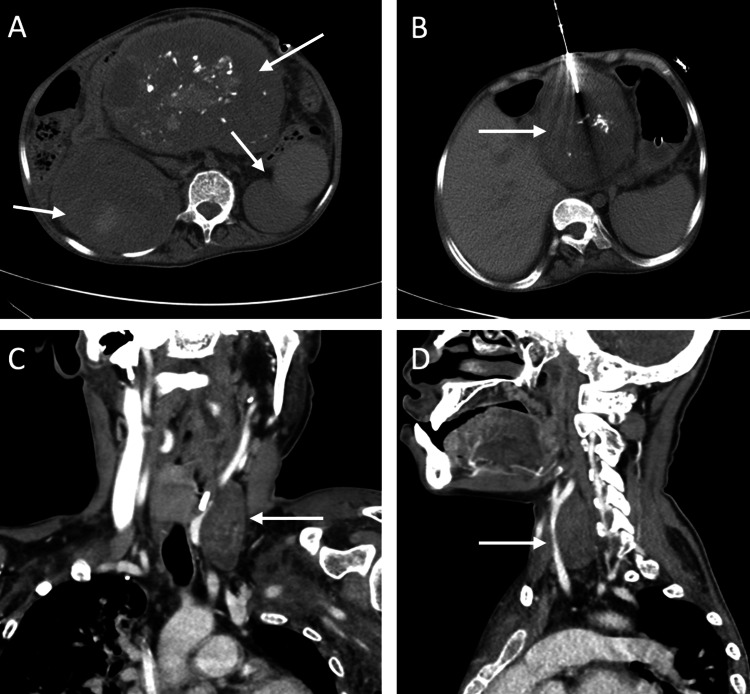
(A) A contrast-enhanced CT scan demonstrates heterogeneous mesenteric and perirenal masses, with the mesenteric mass showing calcifications within. (B) CT-guided biopsy confirming calcifications within the mesenteric mass. (C) Coronal neck CT with intravenous contrast demonstrates a left carotid mass abutting and displacing the left common carotid artery and causing a mass effect upon the left internal jugular vein. (D) Sagittal neck CT with intravenous contrast demonstrates a left carotid mass abutting and displacing the left common carotid artery and causing a mass effect upon the left internal jugular vein.
